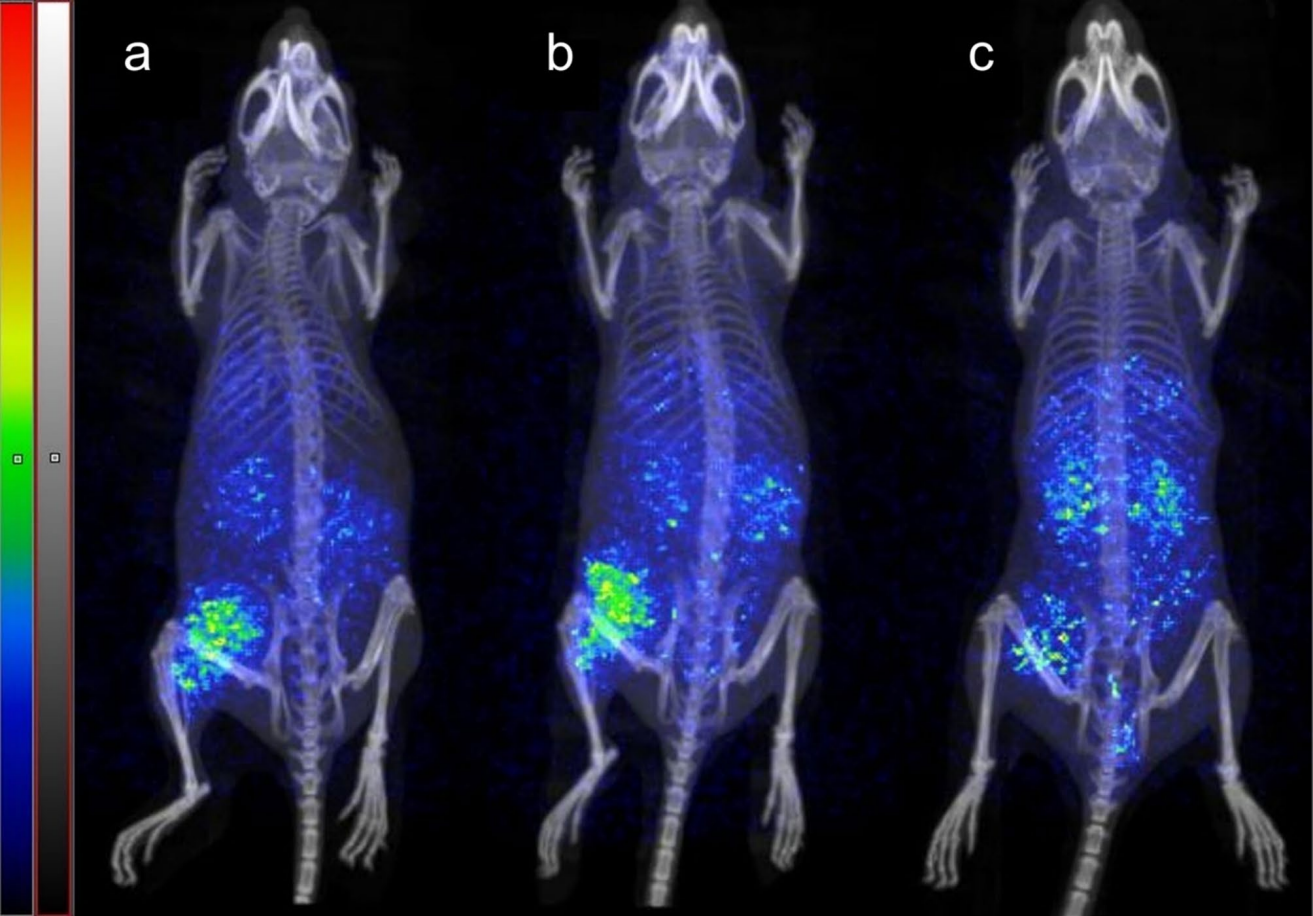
PET/CT scans of (a) [^68^Ga]Ga-NOTA-*o*ET-RM26, (b) [^68^Ga]Ga-NOTA-*o*MA-RM26 and (c) [^68^Ga]Ga-NOTA-*m*MA-RM26. Images are presented in maximal intensity of full body projections on the same scale (minimum: 0 kBq/mL, maximum: 240 kBq/mL)
